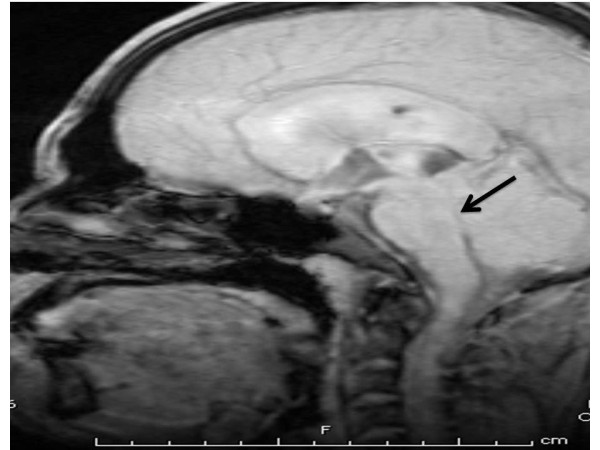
Midsagittal T1-weighted MRI of his head showing hydrocephalus with stenosis of aqueduct (*arrow*).
